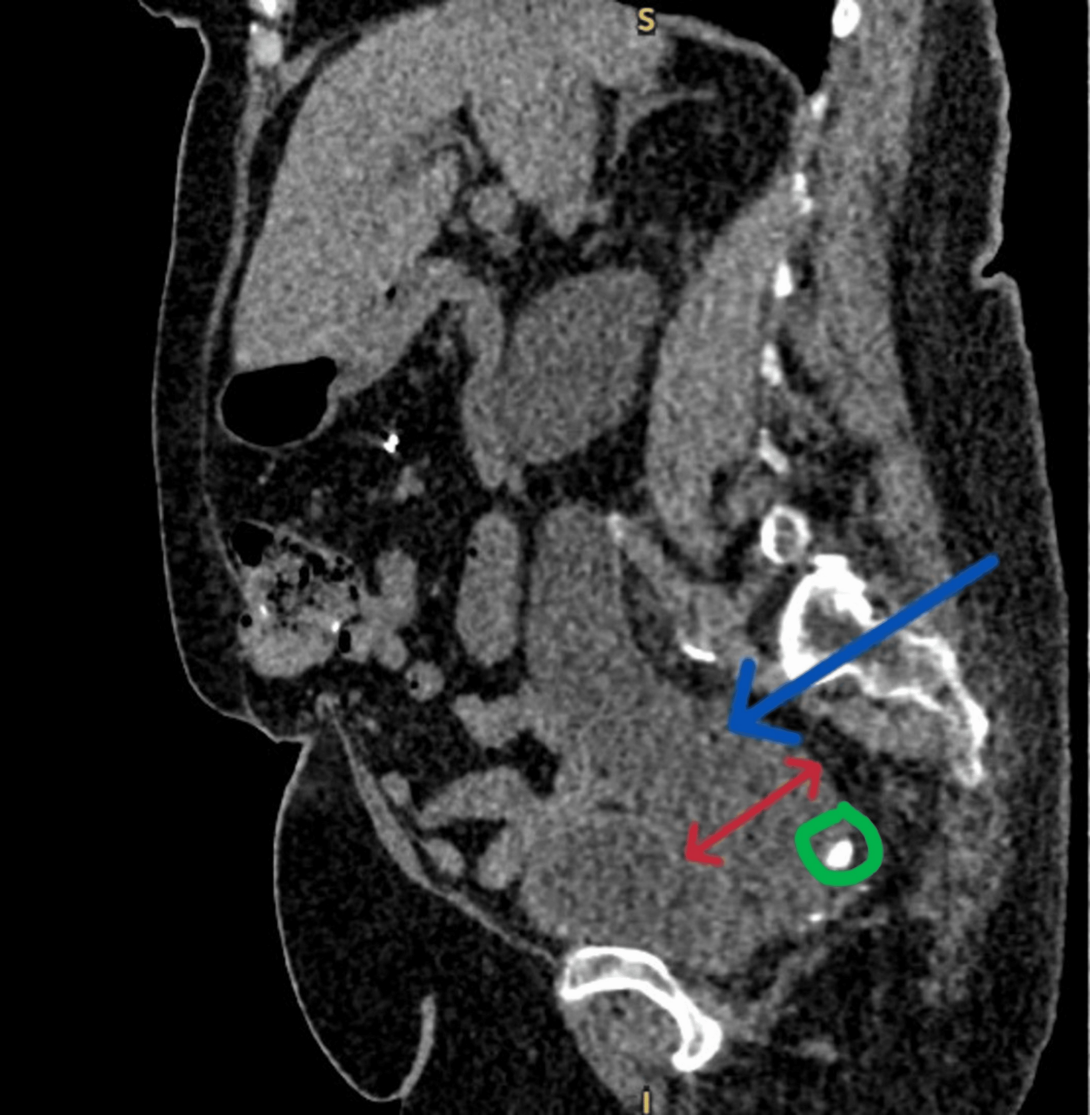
CT sagittal imaging showing a stone in the right distal ureter The blue arrow shows a dilated ureter, while the red arrow indicates the widest ureteric diameter measuring 5.26 cm. The green circle shows the radiopaque stone.
